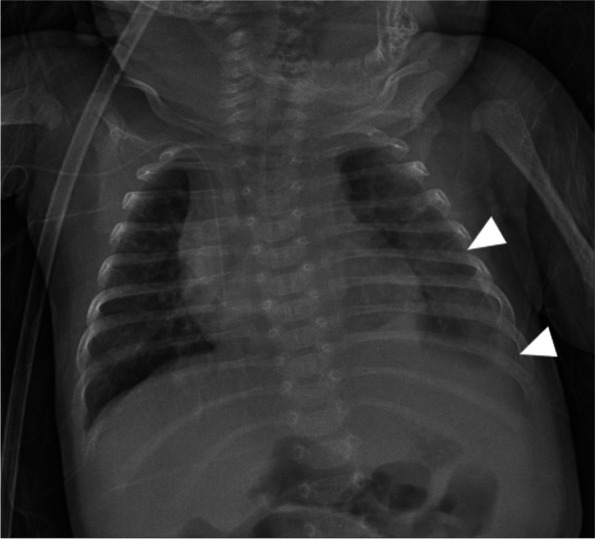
Chest x-ray shows accumulation of left-sided pleural effusions with decreased left lung volume
